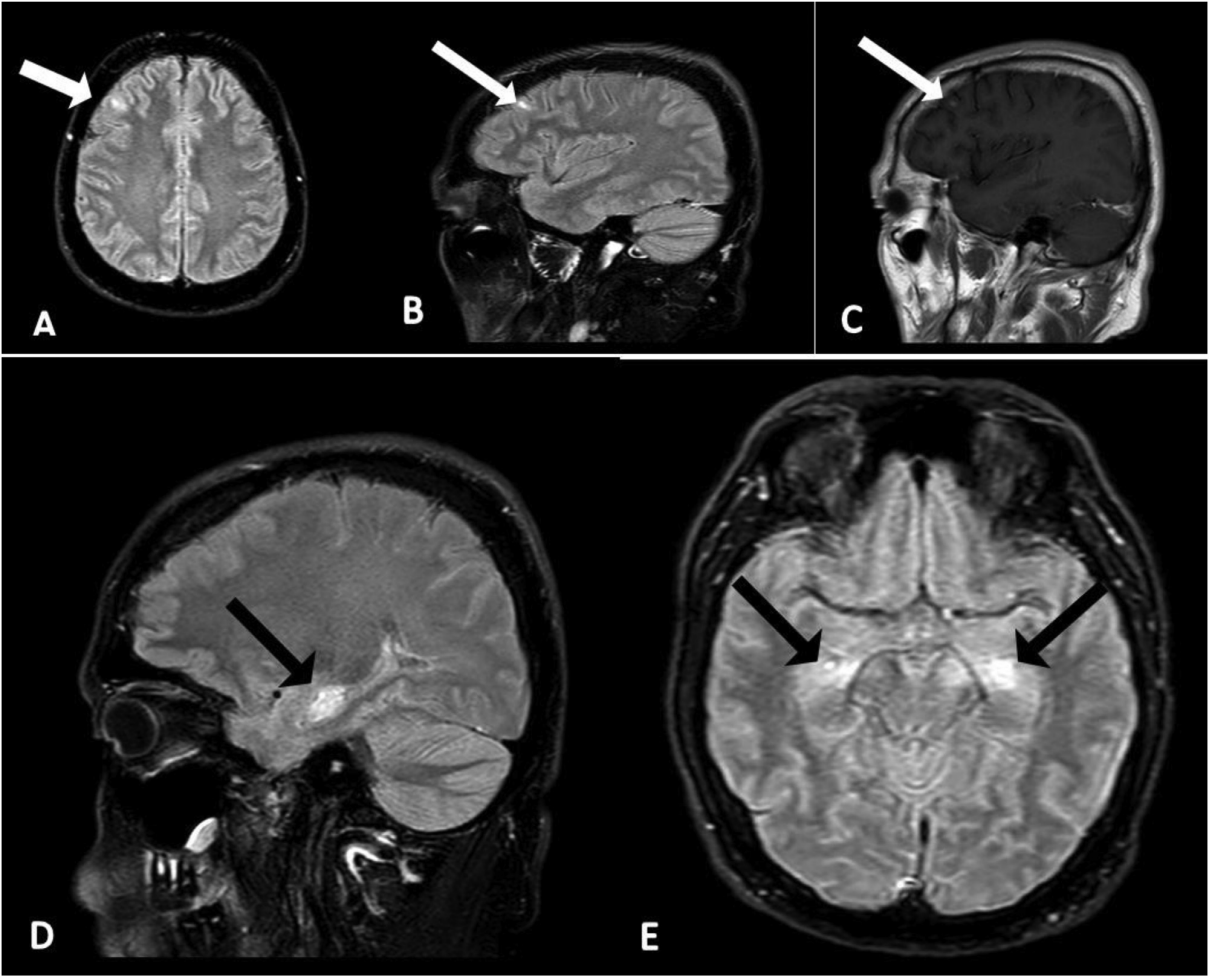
(A,B) Axial and sagittal FLAIR images demonstrate high signal focus in subcortical part of RT frontal lobe. (C) Sagittal postcontrast T1 image shows enhancement )white arrow). (D,E) Sagittal and axial FLAIR images have increase signal of bilateral mesial temporal lobes (black arrows).
